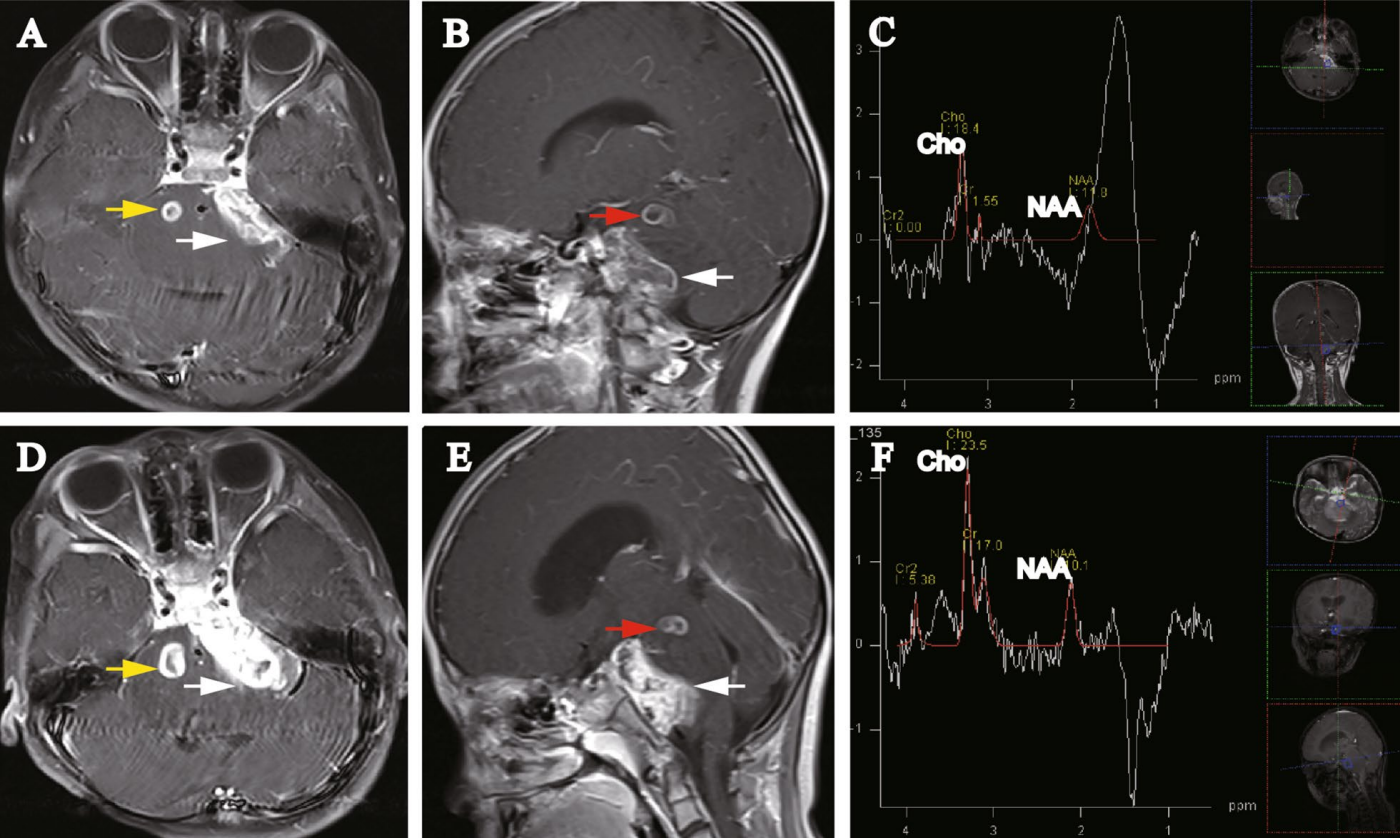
Images of repeated MRI on Dec 11, 2015 and Jan 12, 2016 for the 5-year-old girl with multiple posterior fossa lesions. A Axial gadolinium-enhanced MRI (Dec 11, 2015) displays the occurrence of a new lesion (0.8 cm × 0.9 cm, *yellow arrow*). The *left* CPA lesion is enlarged and extended along the cistern of the CPA (3.1 cm × 1.2 cm, *white arrow*). B Sagittal gadolinium-enhanced MRI (Dec 11, 2015) shows that the CPA lesion was enlarged and extended along the cistern of CPA (*white arrow*) and the *upper left* pontine lesion was enlarged (*red arrow*). C MRS (Dec 11, 2015) shows increased Cho and decreased NAA expression. The Cho:NAA ratio was also significantly increased. D Axial gadolinium-enhanced MRI (Jan 12, 2016) displays that both the CPA lesion (*white arrow*) and the new lesion in the *right side* of the pons are enlarged (1.1 cm × 1.3 cm, *yellow arrow*). The CPA lesion is extended along the cistern of the CPA. E Sagittal gadolinium-enhanced MRI (Jan 12, 2016) shows that both the CPA lesion (*white arrow*) and *upper left* pontine lesion (*red arrow*) were enlarged. F MRS (Jan 12, 2016) shows further increased Cho and decreased NAA expression. The Cho:NAA ratio was 2.33
